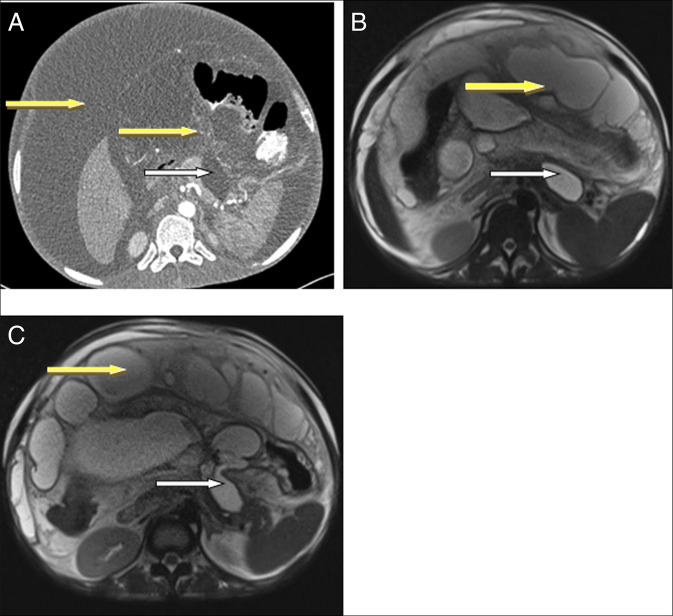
CECT (A) and T2-weighted MRI axial images (B and C) showing cystic pancreatic lesion arising from pancreatic body and tail (white arrows). Omental thickening and multiloculated ascites (yellow arrow). There were no pancreatic ductal dilatation, calcifications, and peripancreatic lymphadenopathy. There were no vascular involvement nor invasion. CECT, contrasted enhanced computed tomography; MRI, magnetic resonance imaging.
